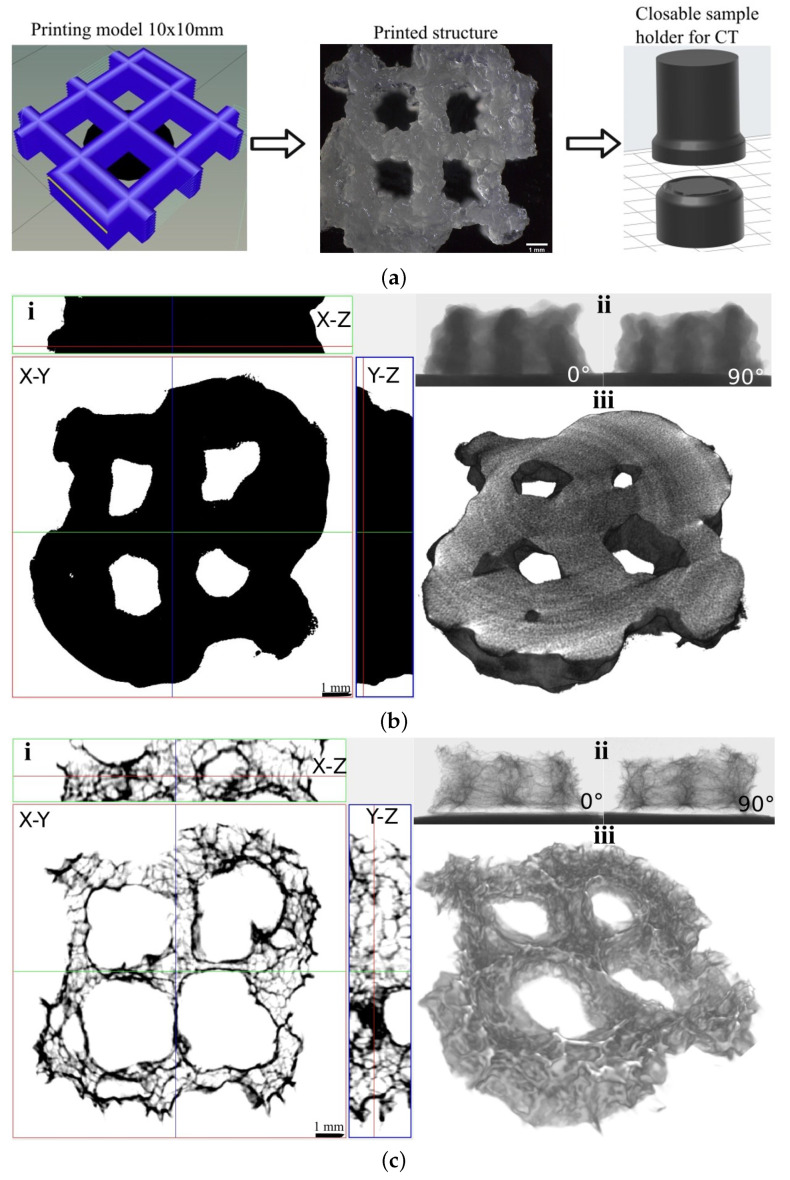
CT analysis of printed structures; (a) Scheme of sample preparation, (b) As-printed structure, (c) Lyophilized structure: i—2D cross-sections in respective planes, ii—X-ray adsorption for either 0${}^{\circ }$ or 90${}^{\circ }$, and iii—3D model.
